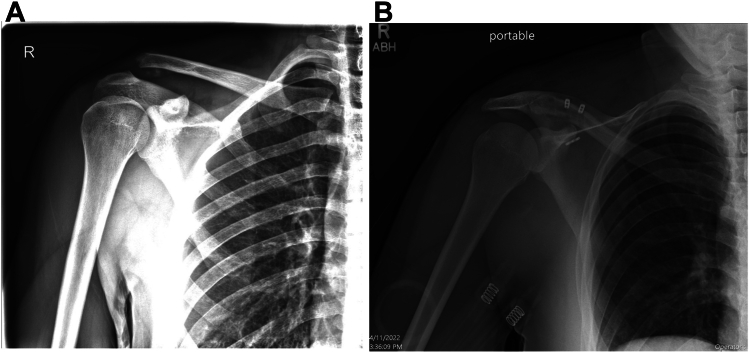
(A) Radiographs demonstrating a right-type III AC joint separation. (B) Radiographs demonstrating maintenance of AC joint reduction. *AC*, acromioclavicular.
